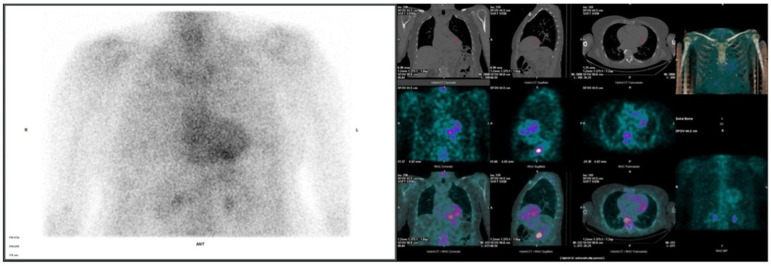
The 99mTc-pyrophosphate (99mTc-PYP) bone scintigraphy with SPECT/CT images; 99mTc-PYP myocardial uptake assessed as Grade 3.
